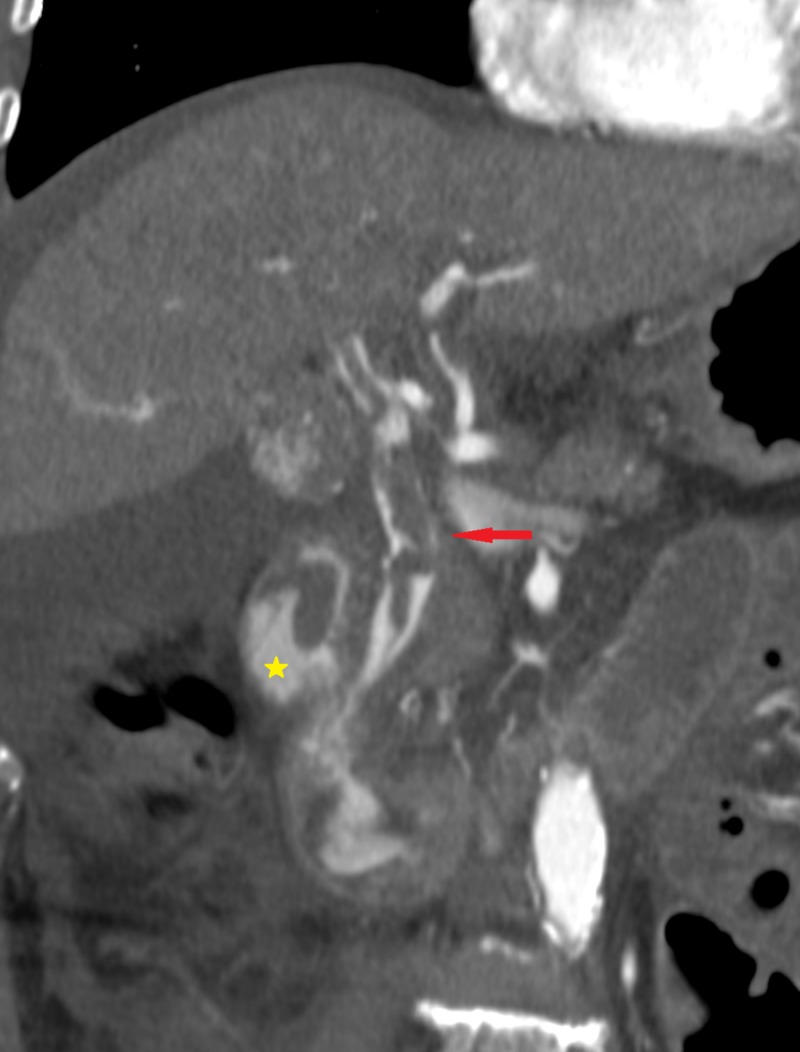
CT angiography Arterial phase images revealed contrast media filling of the biliary tree, the choledocus (red arrow), and the duodenum (yellow star).
